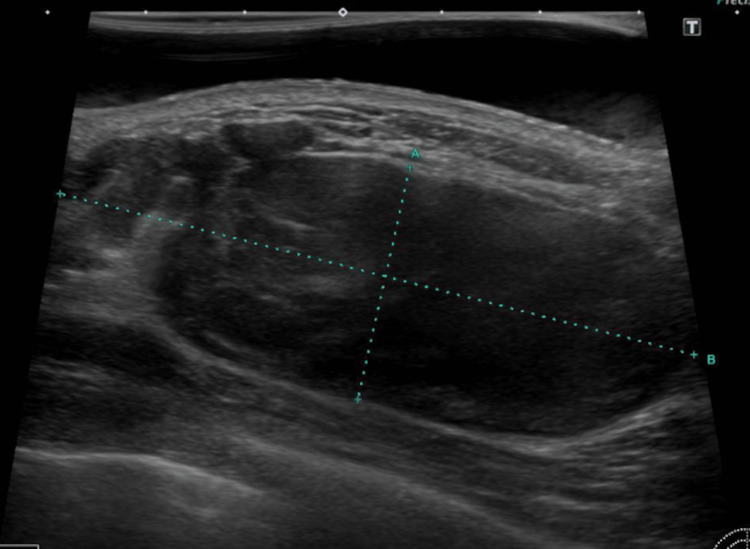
Breast ultrasonography A well-defined hypoechoic tumor sized 24 x 66 x 62 mm was observed in the left upper area
